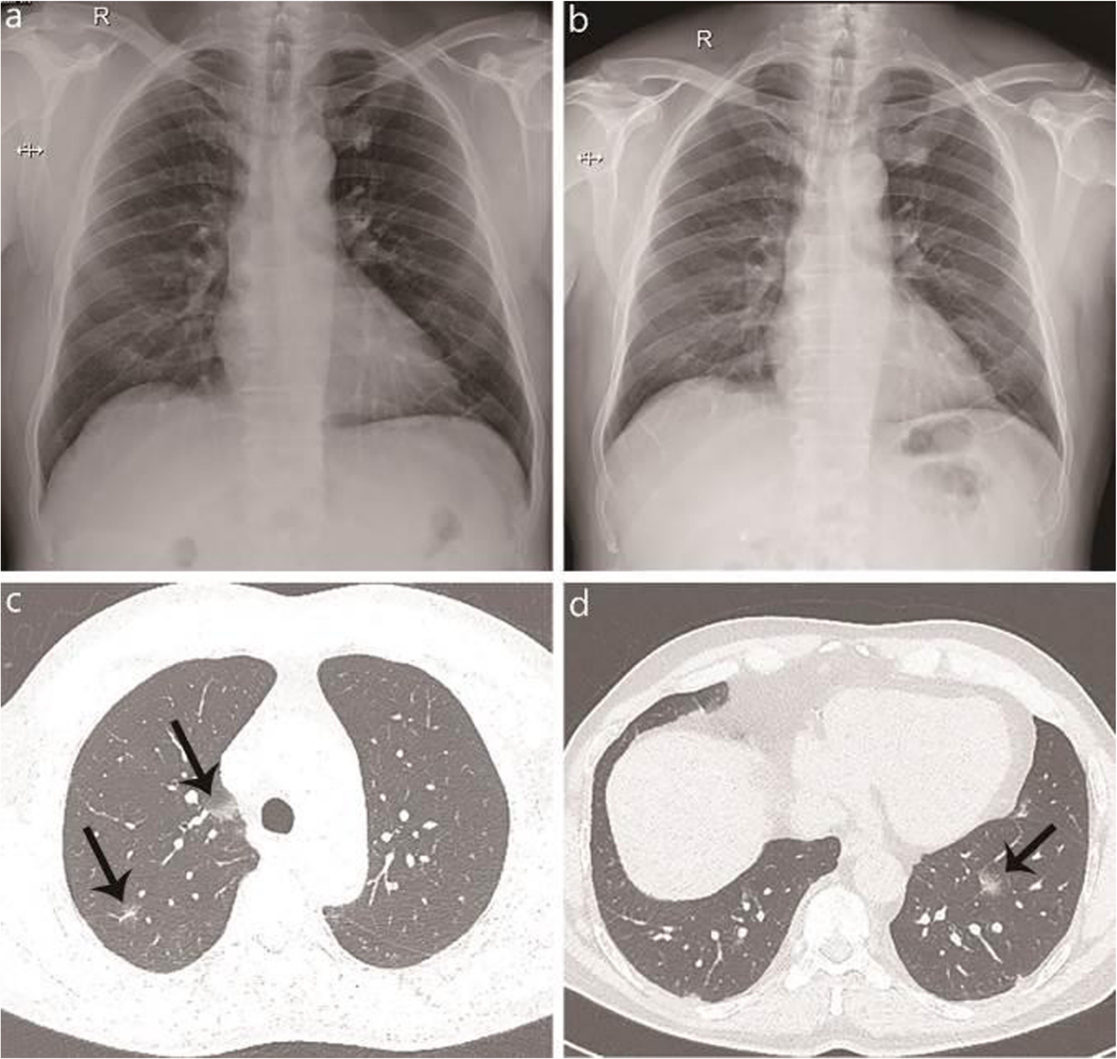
The importance of CT in showing the ground-glass opacities common in disease. a. PA chest radiograph of a 66-year-old male patient in 2016. b. chest radiograph on March 23, 2020. There is no significant difference between the two graphics. The chest radiography is mostly normal, especially during the early disease period. c-d. CT of the same patient on the same date as the second X-ray (March 23, 2020) shows ground-glass opacities (black arrows) in the right middle and bilateral lower lungs and findings were suspicious for COVID-19. The sensitivity of chest radiograph is always lower in demonstrating ground-glass opacities of these sizes and low density
